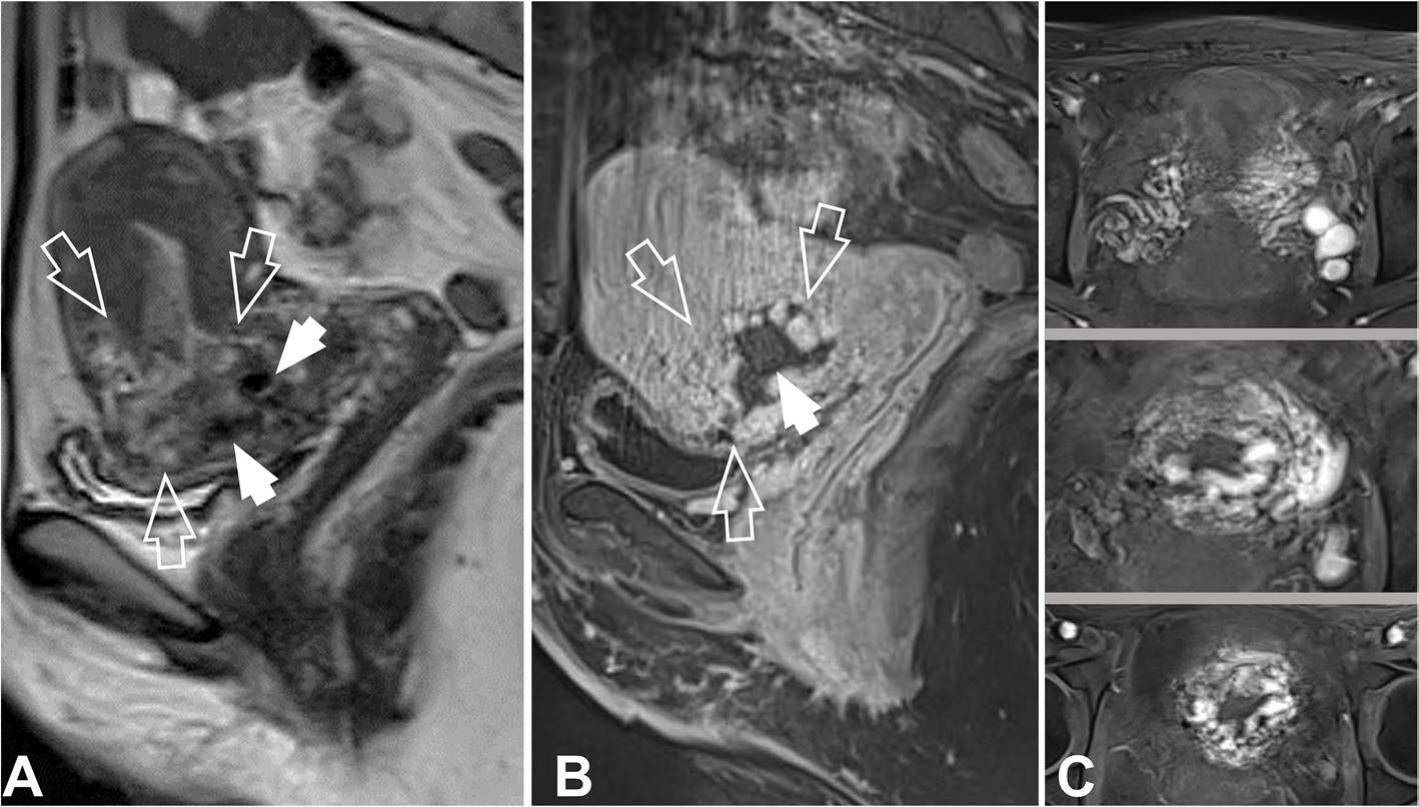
RPOC in a 38-year-old woman with mild secondary PPH (40 days after delivery), characterized as a heterogeneously intense rounded mass, was noted, manifesting a hyperintense signal in T2-weighted imaging and a corresponding hypointense signal in T1-weighted imaging (open arrows in A and B, respectively). Furthermore, multiple regions exhibiting signal void indicative of high-flow vascularization, were observed (arrows in A—B). Contrast-enhanced imaging revealed pronounced arterial phase vascular recruitment and early enhancement (image C)
